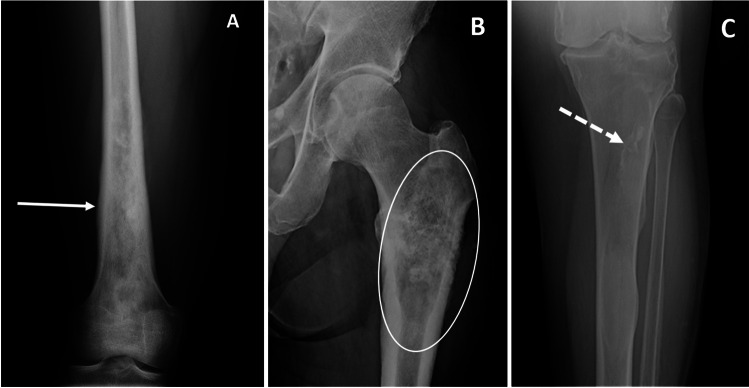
Radiographic examples of osteoid (a arrow — osteosarcoma distal femur), chondroid (b circled area — chondrosarcoma proximal femur), and fibrous (c dotted arrow — fibrous dysplasia proximal tibia) matrix
